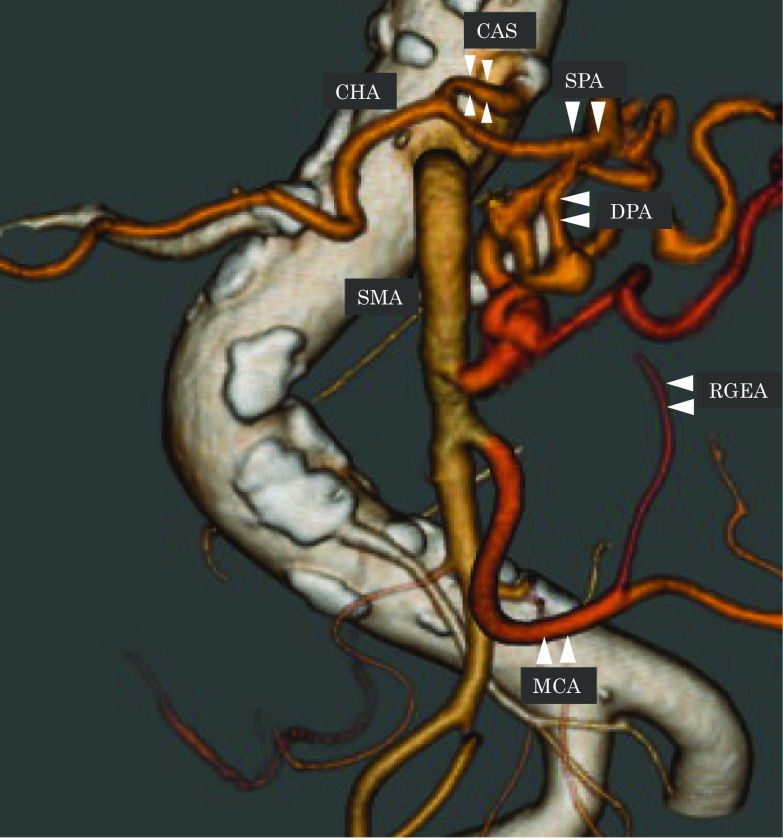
Postoperative MDCT. Postoperative enhanced CT showed that sufficient inflow was provided from the lt. MCA to the RGEA, and sufficient inflow from the SMA to the SPA via the DPA
